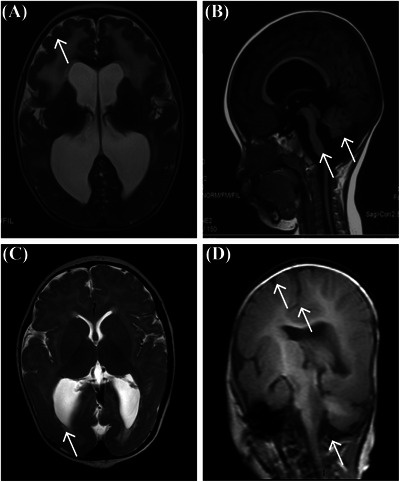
Cerebellar and brainstem abnormalities on brain MRI in patients with *CRPPA*‐related dystroglycanopathy. Representative images from four patients show consistent patterns of cerebellar malformation. (A) Patient 2, anteriorly enlarged cerebellum; (B) Patient 10, cerebellar tonsillar malformation and brainstem dysplasia; (C) Patient 13, cerebellar cyst; (D) Patient 11, reduced cerebellar hemispheres and brainstem dysplasia. White arrows indicate the representative abnormalities. *CRPPA*, CDP‐L‐ribitol pyrophosphorylase A; MRI, magnetic resonance imaging.
